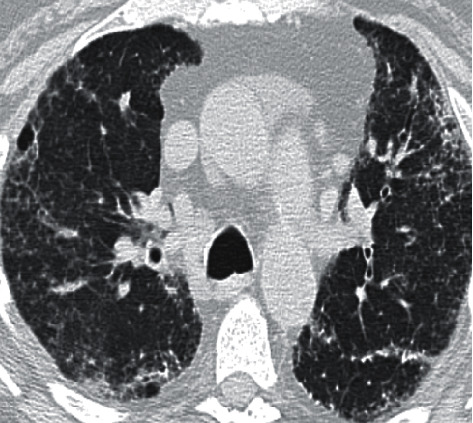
A 72-year old man referred to MDD meeting with a diagnosis of sarcoidosis. HRCT pattern favored chronic HP; however, the case was deemed unclassifiable after multidisciplinary discussion as no exposure was elicited and due to the high clinical probability of IPF. The patient was referred to surgical lung biopsy that demonstrated a usual interstitial pneumonia (UIP) pattern and led to a diagnosis of IPF. The patient was subsequently started on antifibrotic treatment.
